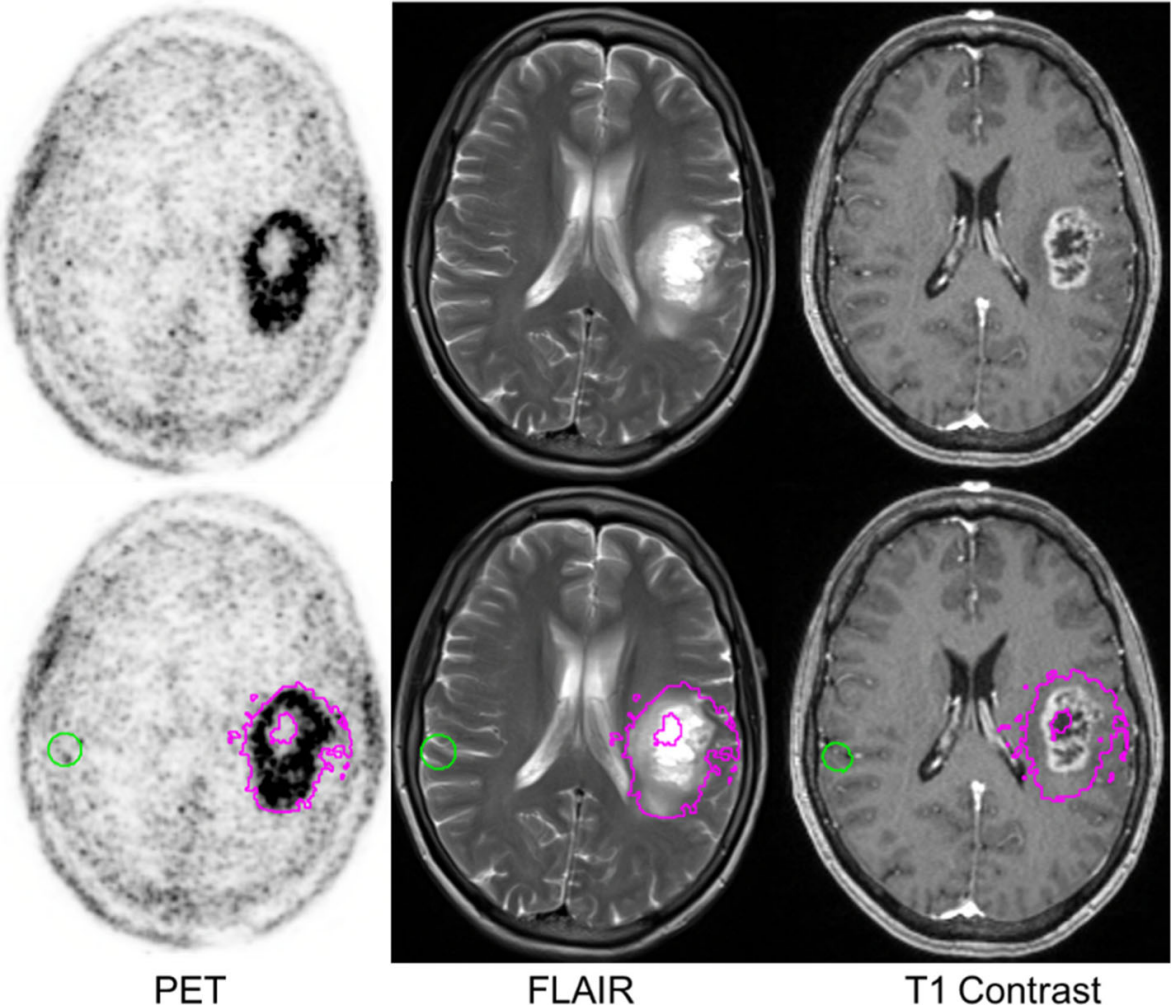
Fluciclovine PET at 30 min post-injection of glioblastoma fused with regions overlaid on MRI. Green sphere is the area of normal brain fluciclovine uptake. Magenta area is the metabolic tumor uptake defined as 1.3* contralateral normal brain uptake (TB_mean1.3_)
